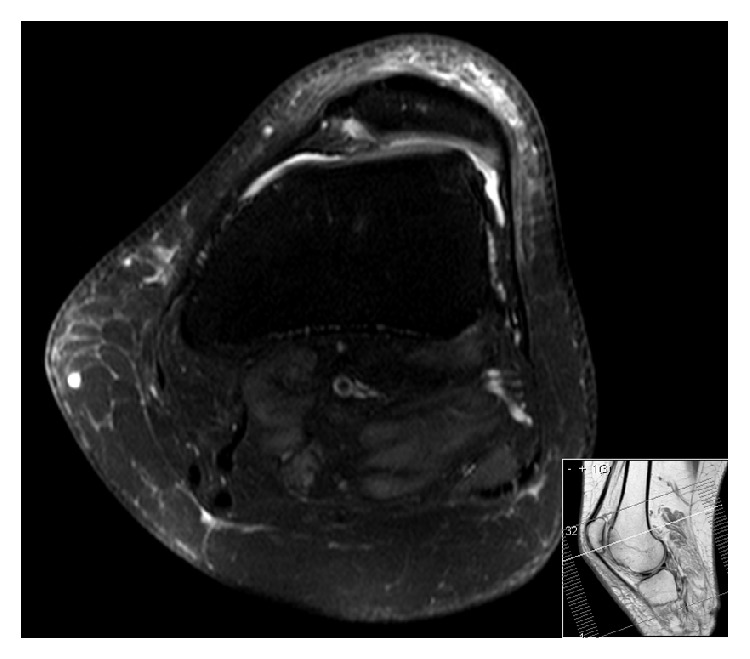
MRI T2-weighted axial image showing a Dejour type C trochlea with lateral condyle convexity and medial condyle hypoplasia.
